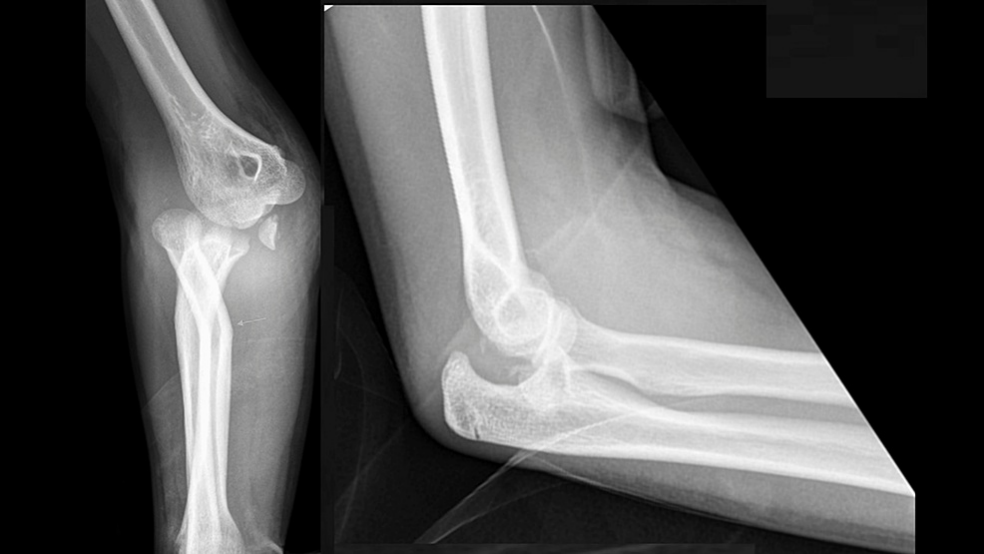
Radiographs of the elbow showing medial dislocation, medial epicondyle fracture, and non-displaced radial shaft fracture.
